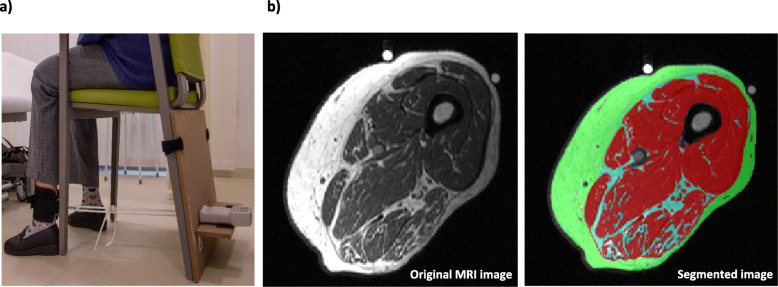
a Maximal isometric knee extension strength measurement and b A sample of single-slice mid-thigh MRI image of a 68-year-old female, mobile patient before and after segmentation. Structures in red: muscle, green: subcutaneous fat, blue: intermuscular fat
